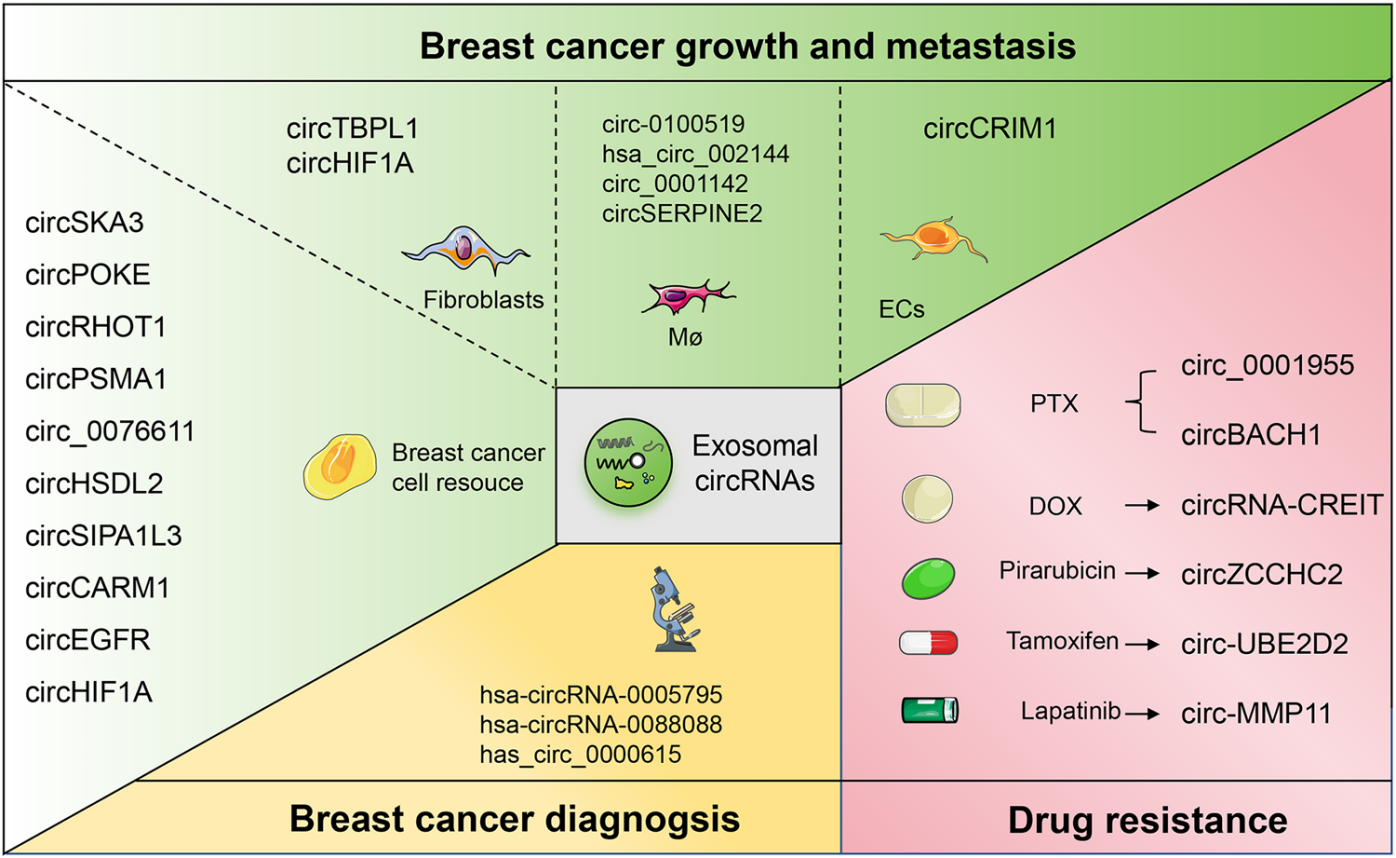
The multifaceted roles of exosomal circRNAs in breast cancer progression, diagnosis, and drug resistance. In cancer progression, circRNAs such as circSKA3, circPOKE, and circRHOT1 enhance tumor invasion, metastasis, and proliferation by modulating pathways like the USP10-Snail axis and miR-204-5p/PRMT5 axis. CircPSMA1 and circHSDL2 contribute to tumorigenesis and migration through interactions with key cellular pathways, including the miR-637/Akt1/β-catenin axis. Additionally, circSIPA1L3 and circCARM1 are involved in metabolic reprogramming, promoting glycolysis and tumor growth. In diagnosis application, circRNAs such as hsa-circRNA-0005795 and has_circ_0000615 emerge as promising biomarkers due to their differential expression in breast cancer patients. For drug resistance, circRNA-CREIT and circBACH1 play pivotal roles in modulating the sensitivity of breast cancer cells to treatments like paclitaxel and doxorubicin, impacting pathways such as the miR-217/G3BP2 axis. These circRNAs are integral to the modulation of tumor behavior, immune regulation, and therapeutic responses, providing valuable insights and targets for innovative breast cancer diagnostics and treatment strategies.
